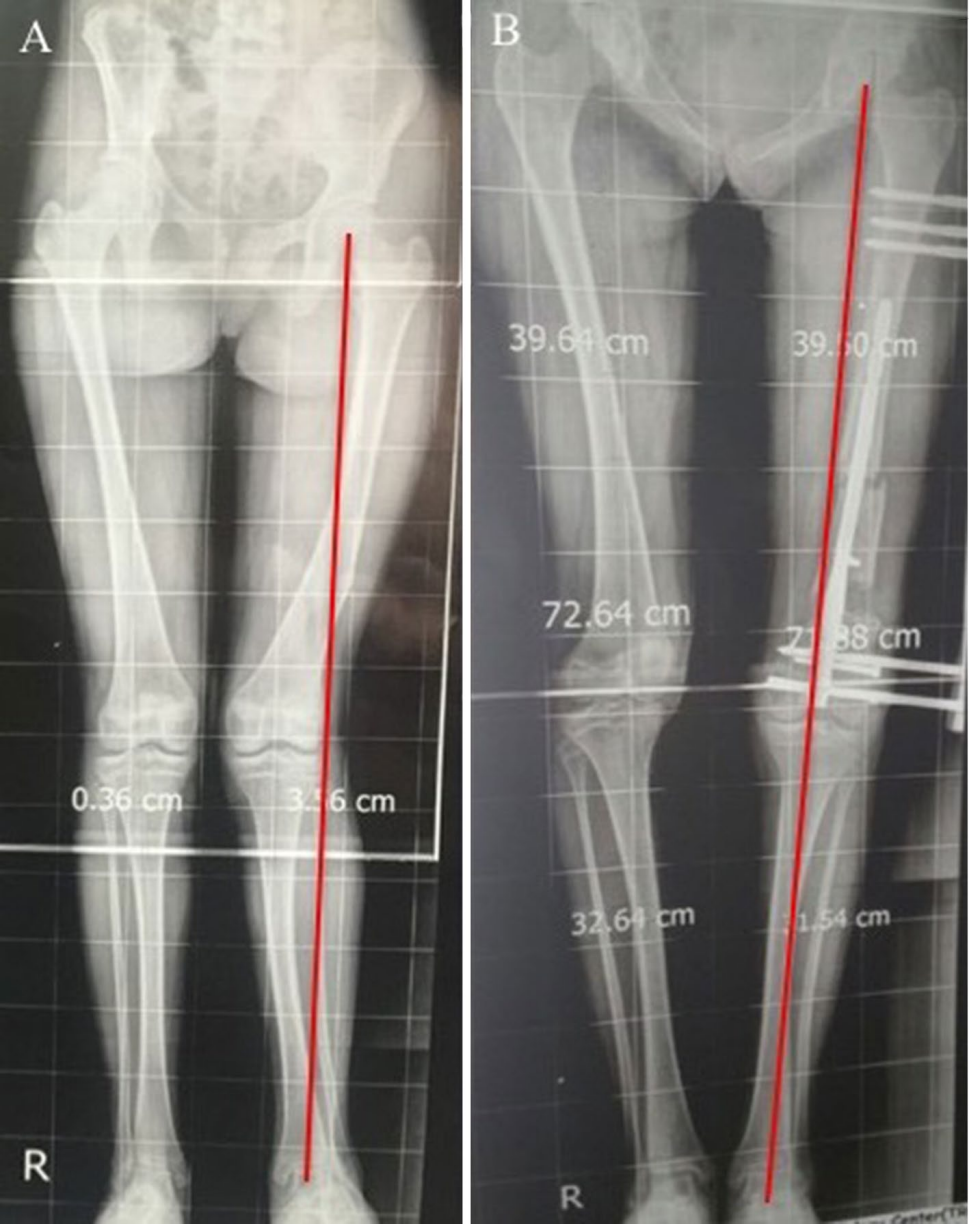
a Pre-operative radiograph showing the combined shortening and valgus malalignment of the left femur, b the reproduction of the planning and achieving normal alignment at the end of lengthening with no residual MAD
